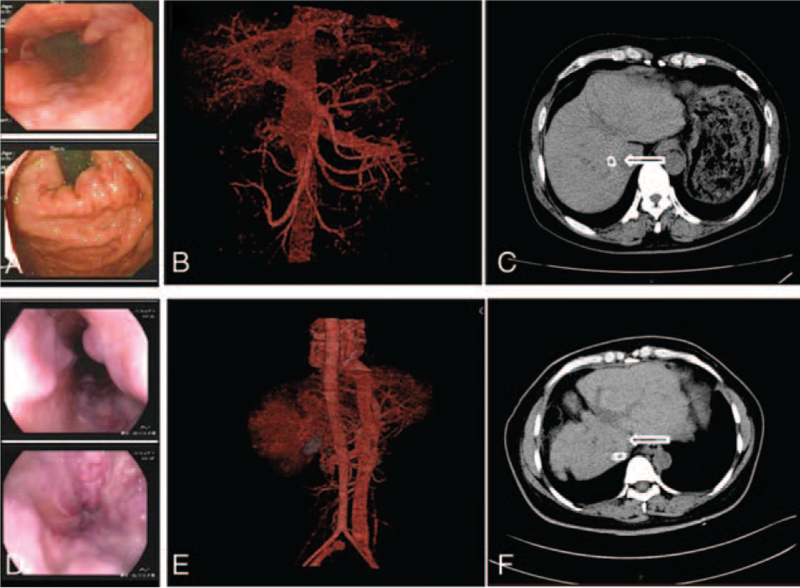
Laboratory examinations of case 1 and case 2. (A,D) preoperative case 1 and case 2 endoscopic esophagus and gastric fundus, (B,E) preoperative abdominal angiography, (C,F) abdominal CT after TIPS. CT = computed tomography, TIPS = transjugular intrahepatic portosystemic shunt.
